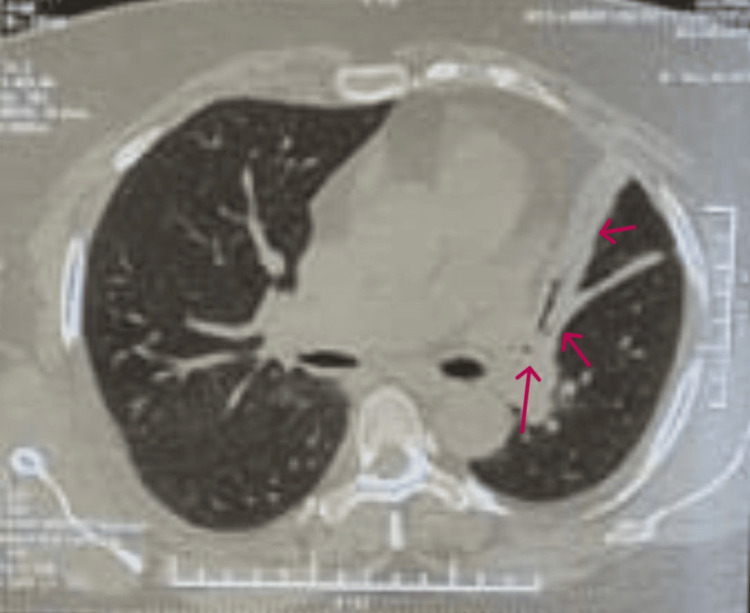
Axial section of the computed tomography of the thorax showing an endoluminal lesion in the left upper lobe with linear atelectasis of the left lingular segment, anterior and posterior segments of the left upper lobe
